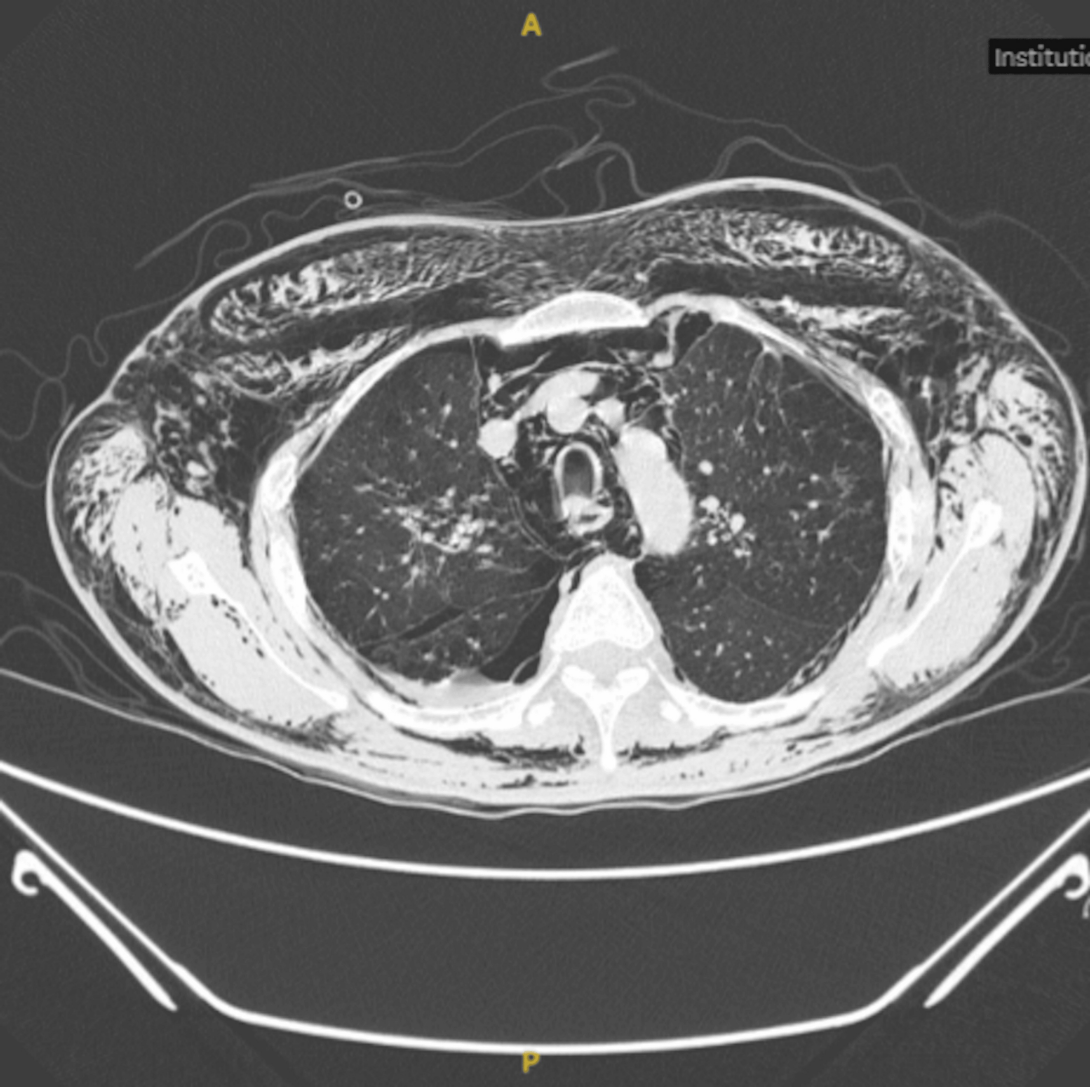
Computed tomography of the thorax (axial view) shows severe bilateral subcutaneous emphysema and pneumomediastinum
